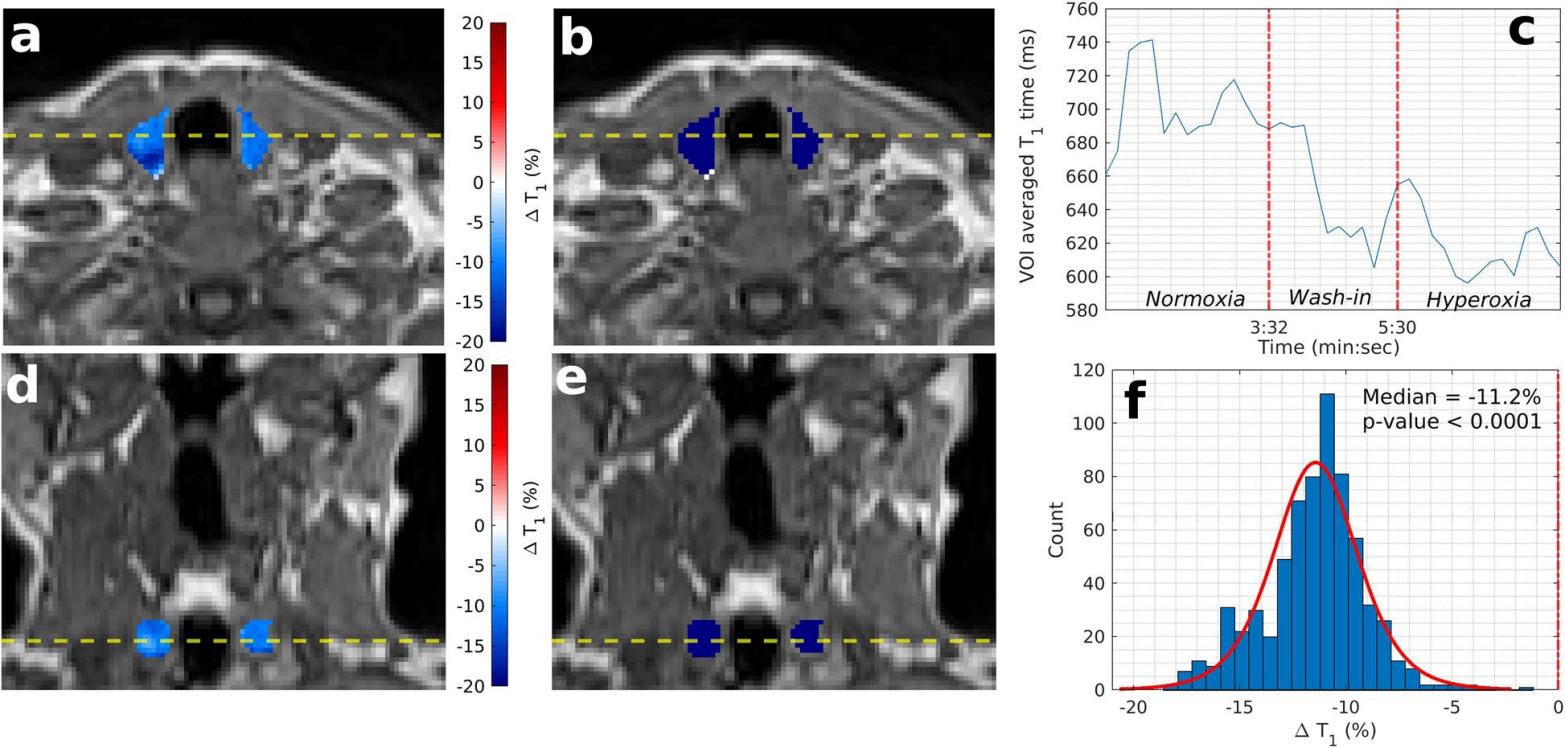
Example OE-MRI parametrical maps of thyroid gland (patient number 5). T1 weighted VIBE image is shown with overlaid parametric ΔT1 map of the thyroid gland (a, d, axial and coronal plane respectively) and overlaid statistical map of ΔT1 times (b, e). Blue colour indicates statistically significant decrease in T1 times, white indicates no statistically significant change, and red indicates statistically significant increasing T1 times. c Time series of T1 times averaged over the entire thyroid VOI. f Histogram of ΔT1 times for the entire thyroid VOI. *OE-MRI* Oxygen-enhanced magnetic resonance imaging, *VIBE* Volumetric interpolated breath-hold examination, *VOI* Volume of interest
